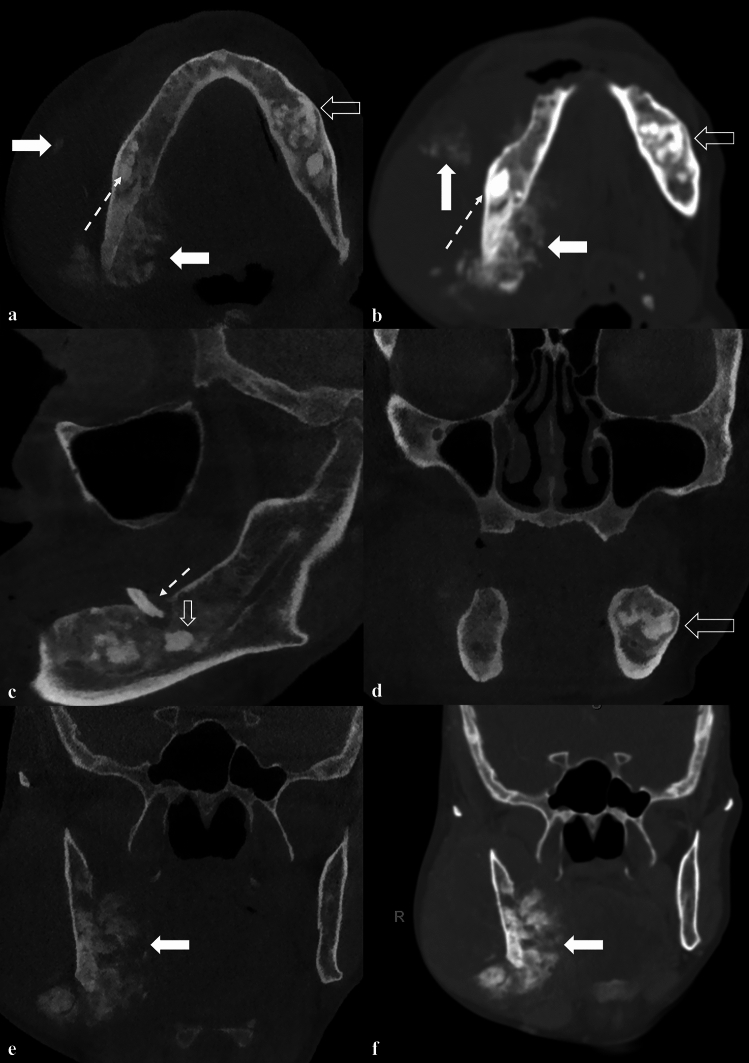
a CBCT axial slice, b CT axial slice; shows bone formation within the lesion at the right mandible (solid arrow). A cotton wool appearance is seen in the right mandible (dashed arrow), closely related to the OS. A mixed density is seen in the left mandible (open arrow). c CBCT sagittal oblique slice shows a root remnant in the left mandible with a periapical low-density (dashed arrow); inferiorly there is a mixed heterogenous lesion. d CBCT coronal slice shows an intermediate heterogenous lesion in the left mandible causing lateral expansion (solid arrow). e CBCT coronal slice, f CT coronal slice; bone formation radiation from the lingual aspect of the right mandible; buccal soft tissue swelling also evident (solid arrows)
